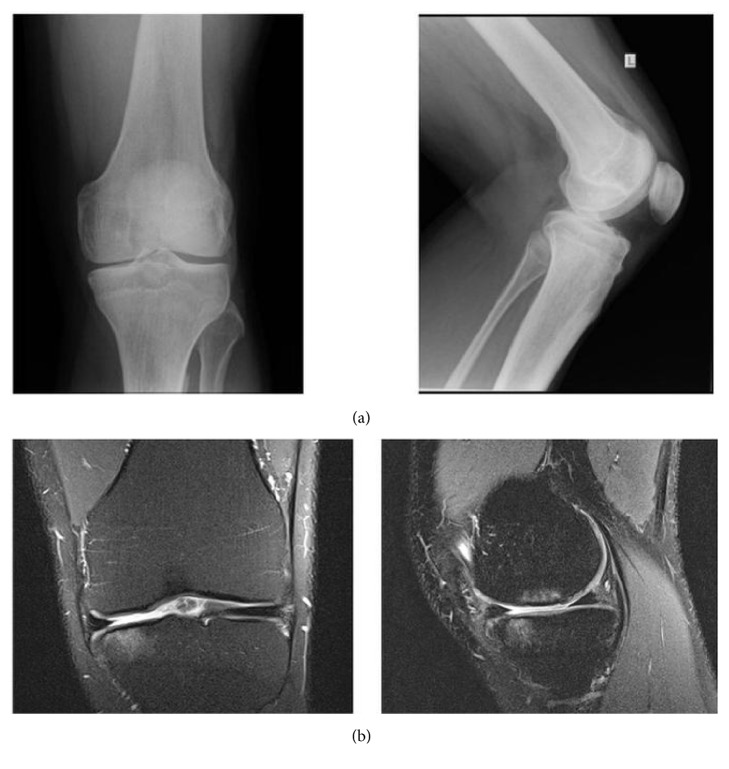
Presurgery anterior and medial radiographs (a) and coronal and sagittal MRIs (b) of the affected left knee.
